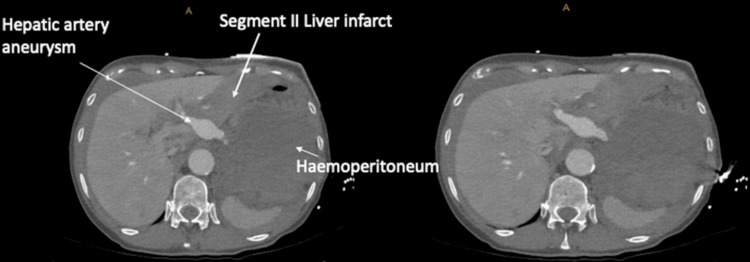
Axial phase of CT aortogram demonstrating ruptured left hepatic artery aneurysm with haemoperitoneum and segment II liver infarct.
